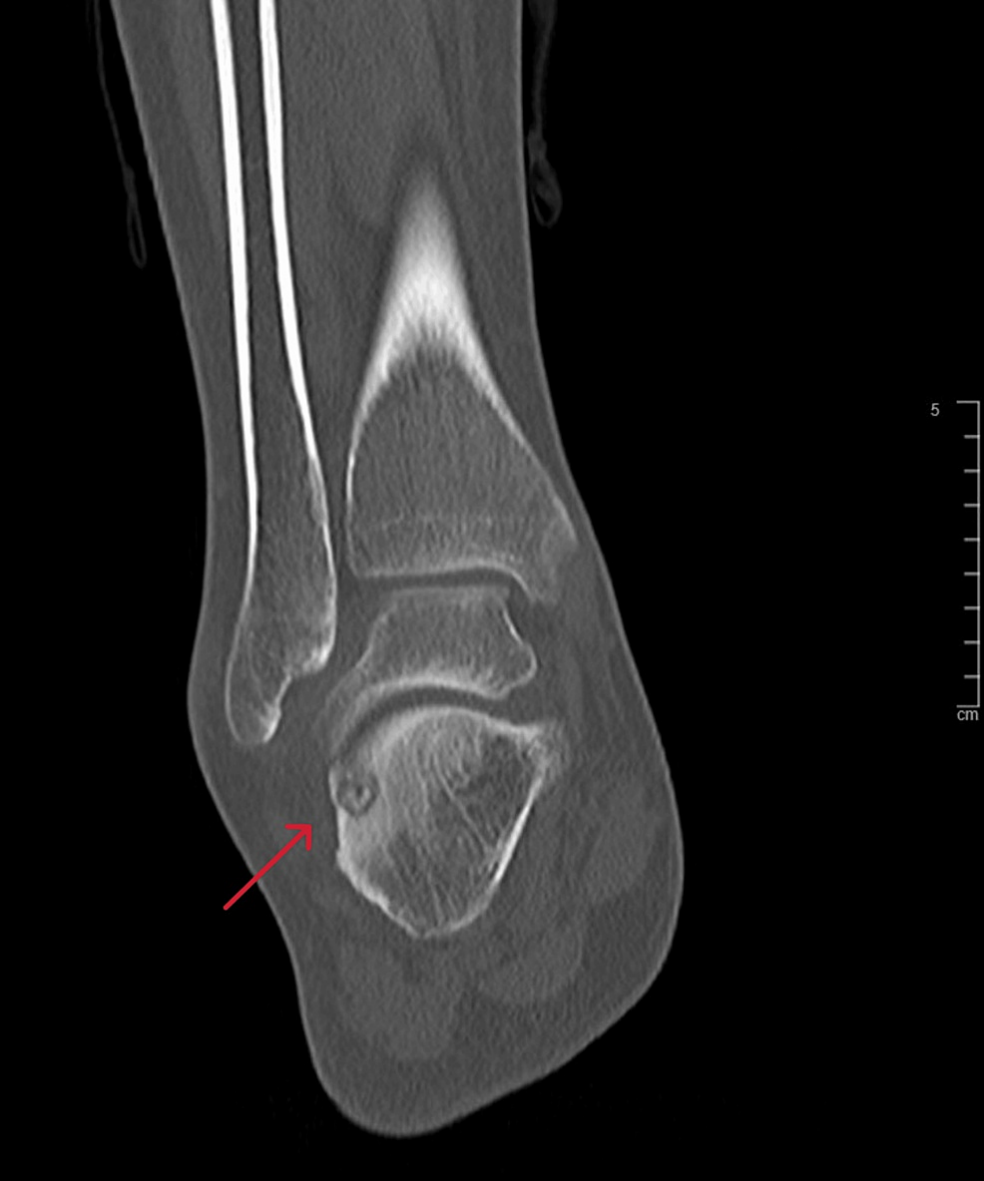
Coronal view CT scan of the right ankle at the time of presentation CT: computed tomography
